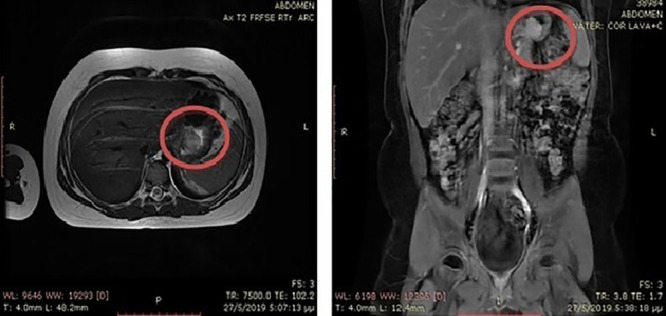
MRI of the thorax and pelvis. MRI = magnetic resonance imaging.
